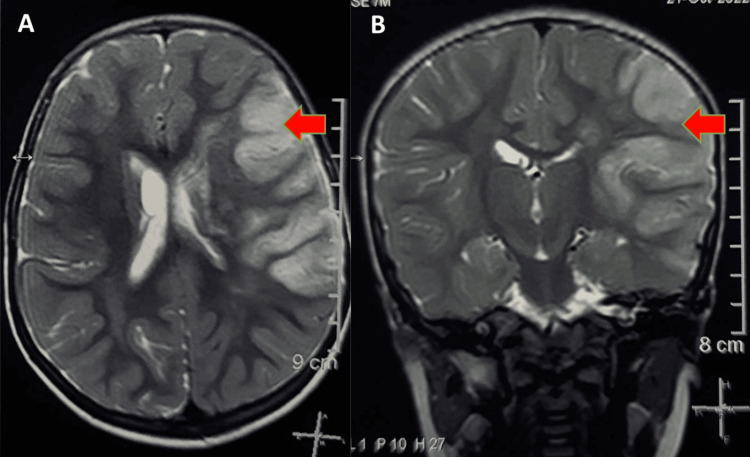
T2 weighted MRI in axial (A) and sagittal (B) section showing hyperintensity in the left middle cerebral artery distribution, that is, left temporal, parietal, frontoparietal, and parietooccipital regions.
